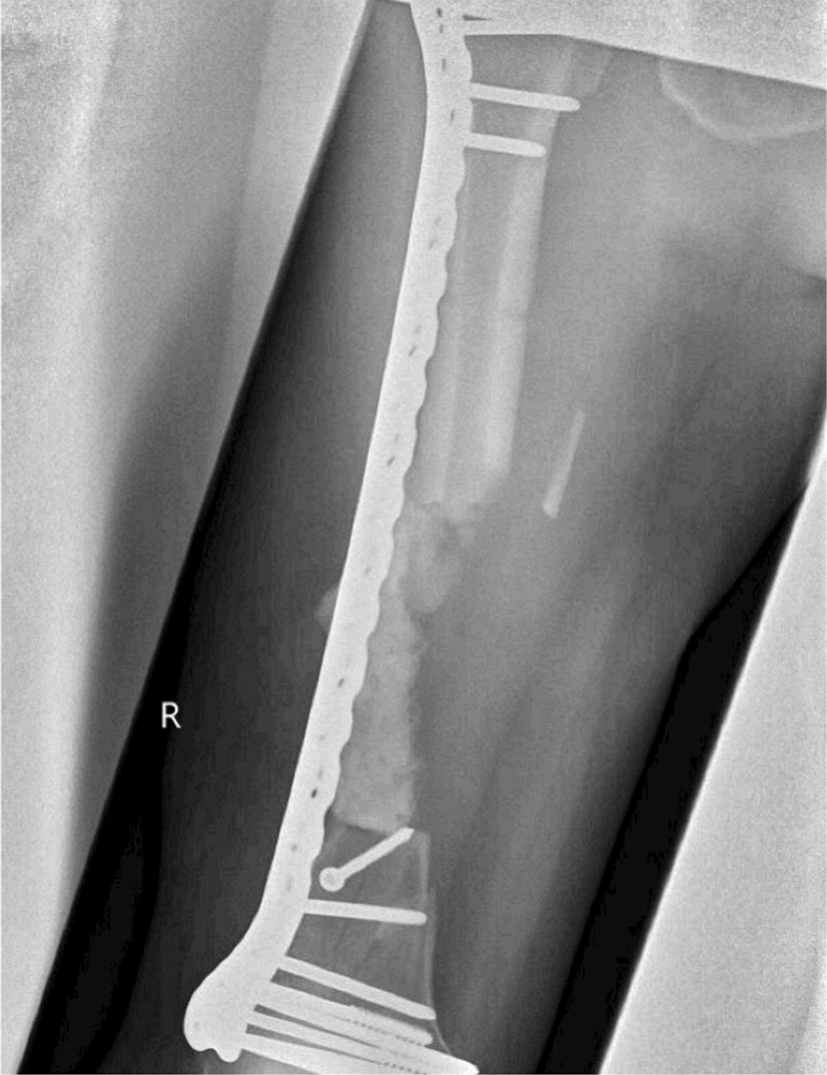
AP radiograph of femur showing the cement spacer and hard callus at the time of surgery
